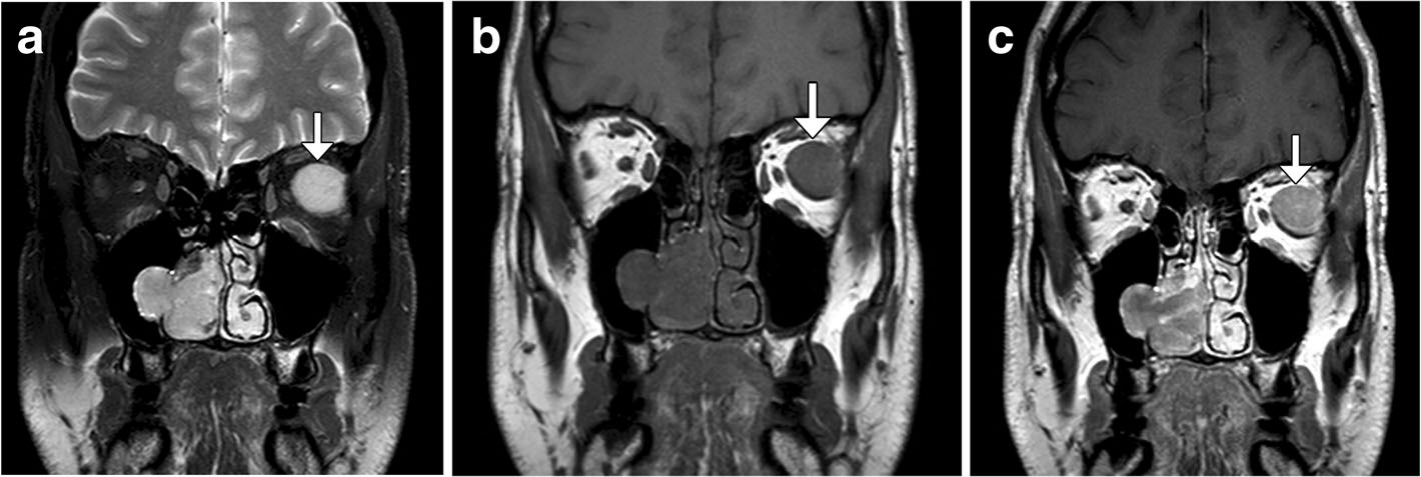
Metastatic mucosal melanoma. Coronal T2-weighted (a), T1-weigthed (b), and post-contrast T1-weighted (c) MR images show am enhancing high T2 and intermediate T1 signal right sinonasal mass with extension into the right maxillary sinus via a secondary ostium and a left lateral rectus metastasis (arrows)
